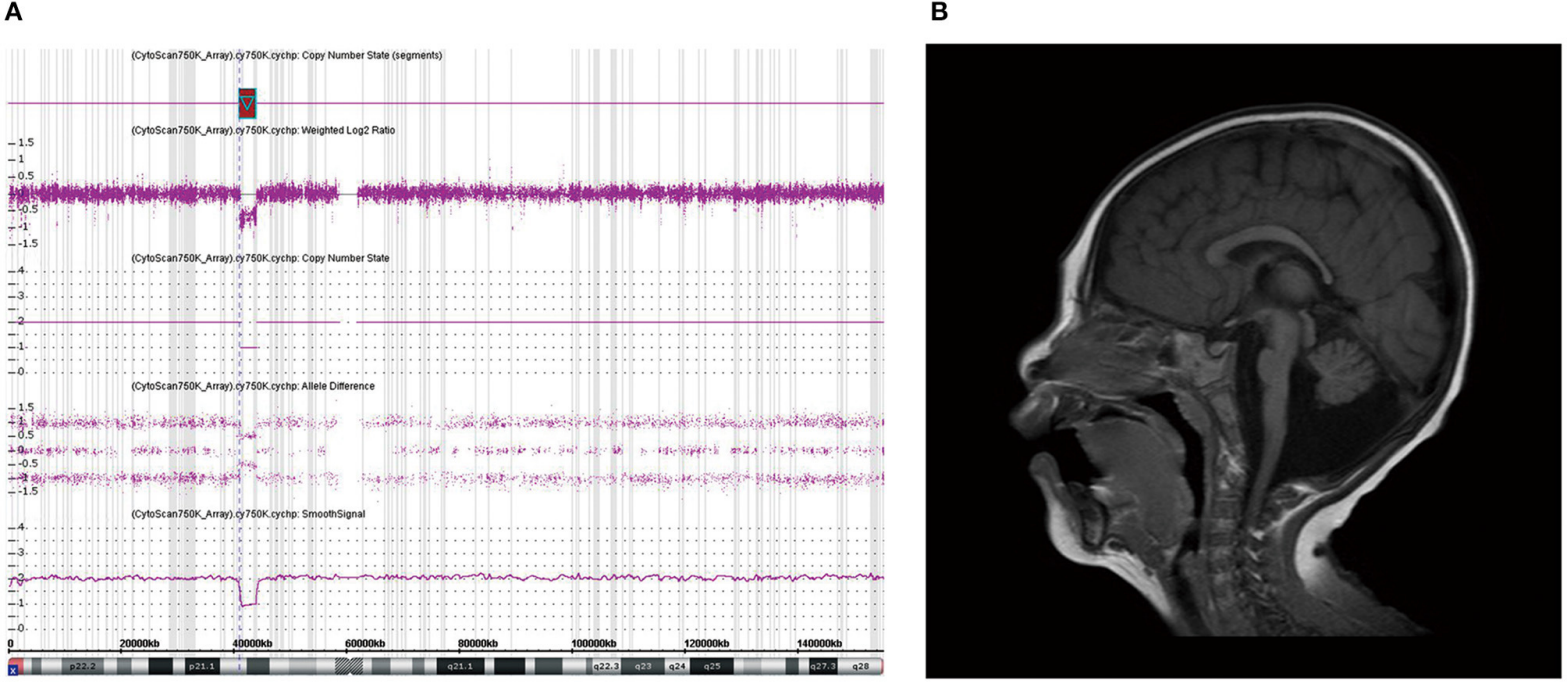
Chromosomal microarray (CMA) and brain magnetic resonance imaging (MRI) of patient M-075. (a) CMA showing a 2.8-Mb deletion at Xp11.4p11.3 containing the *CASK* gene. (b) Brain MRI of patient (M-075) demonstrating the microcephaly with pontine and cerebellar hypoplasia in the T2-weighted sagittal view.
